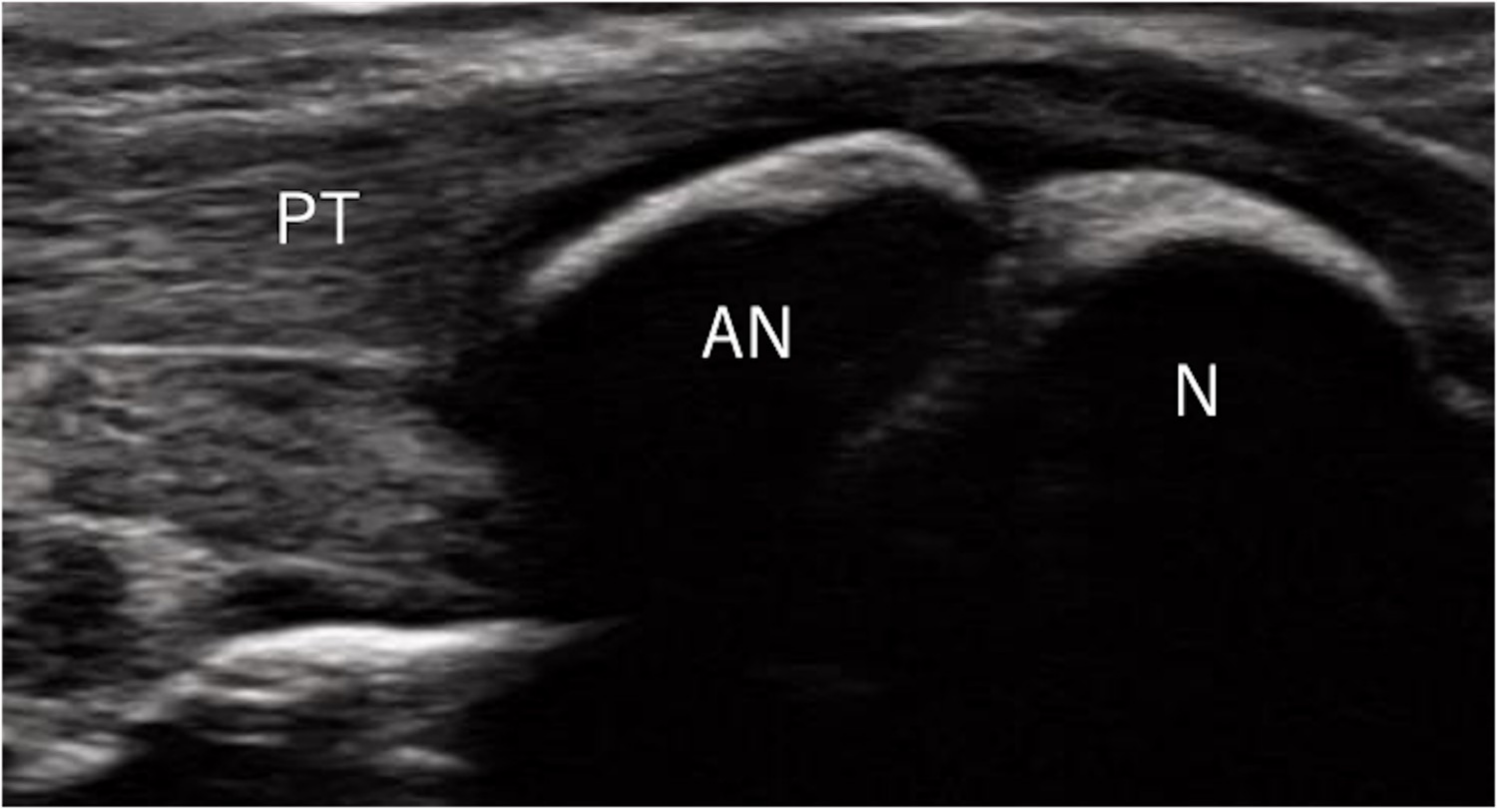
Ultrasonographic findings of the accessory navicular bone. The accessory navicular bone is identified on the proximal side of the navicular bone. Note: PT = posterior tibialis tendon; AN = accessory navicular; N = navicular.
